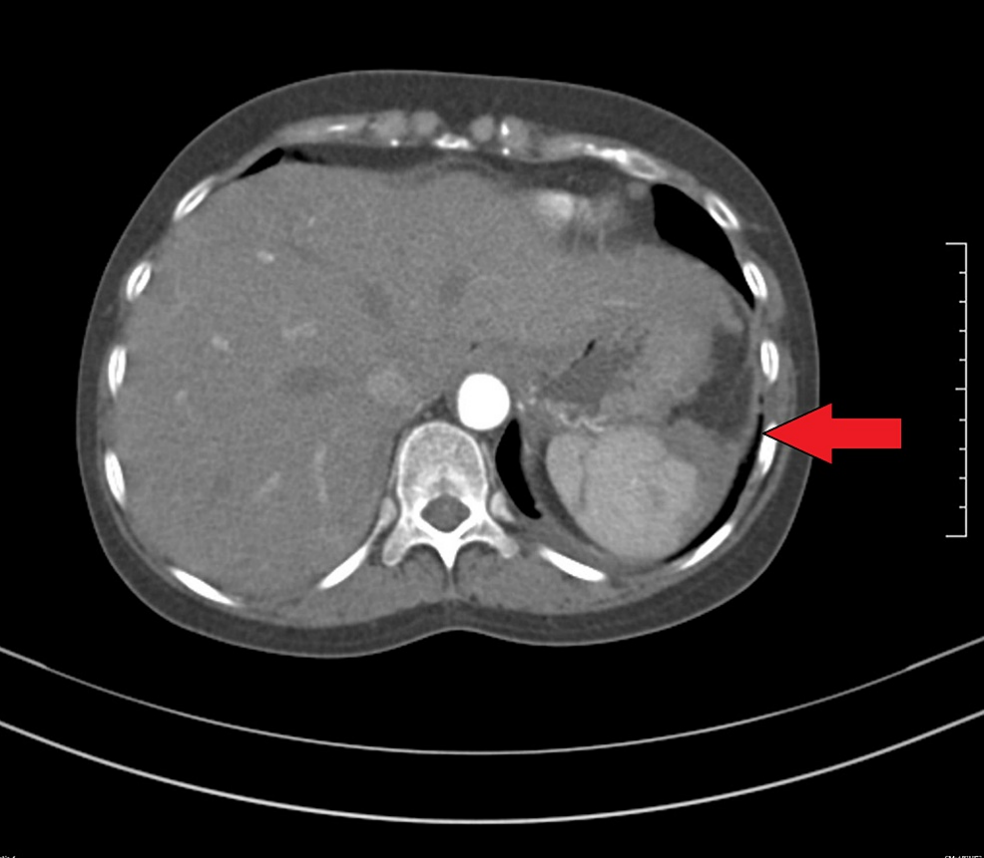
CT abdomen showing significantly resolved splenic abscess six months after treatment.
